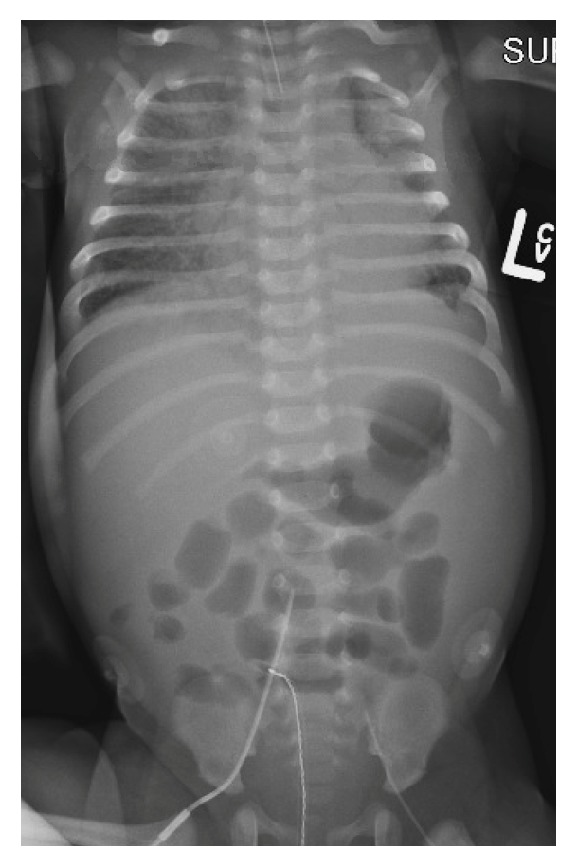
Radiograph of infant with heart failure and mild hypothermia showing central venous catheter overlying L3 vertebral body.
